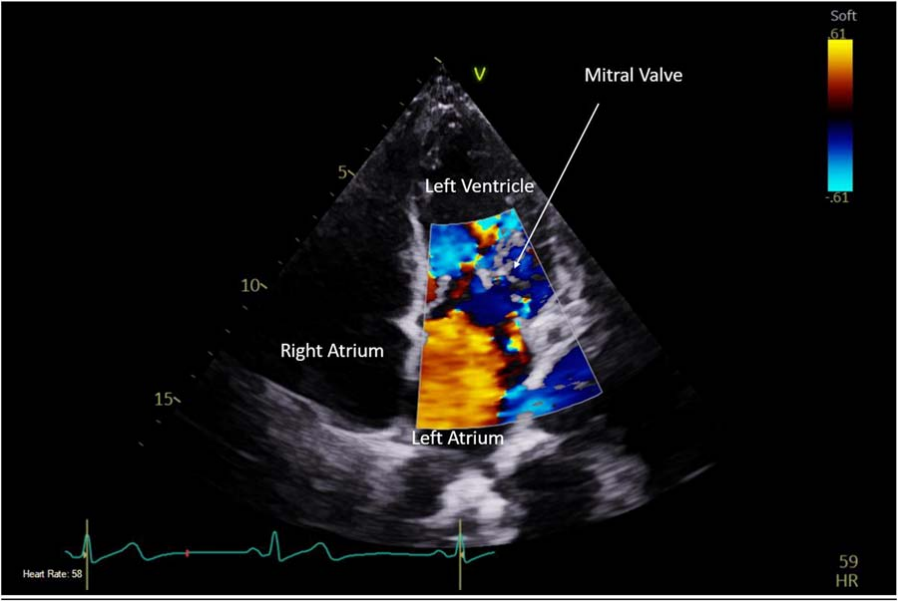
Pre-operative TTE with Doppler depicting mitral valve with vegetation (labelled).
